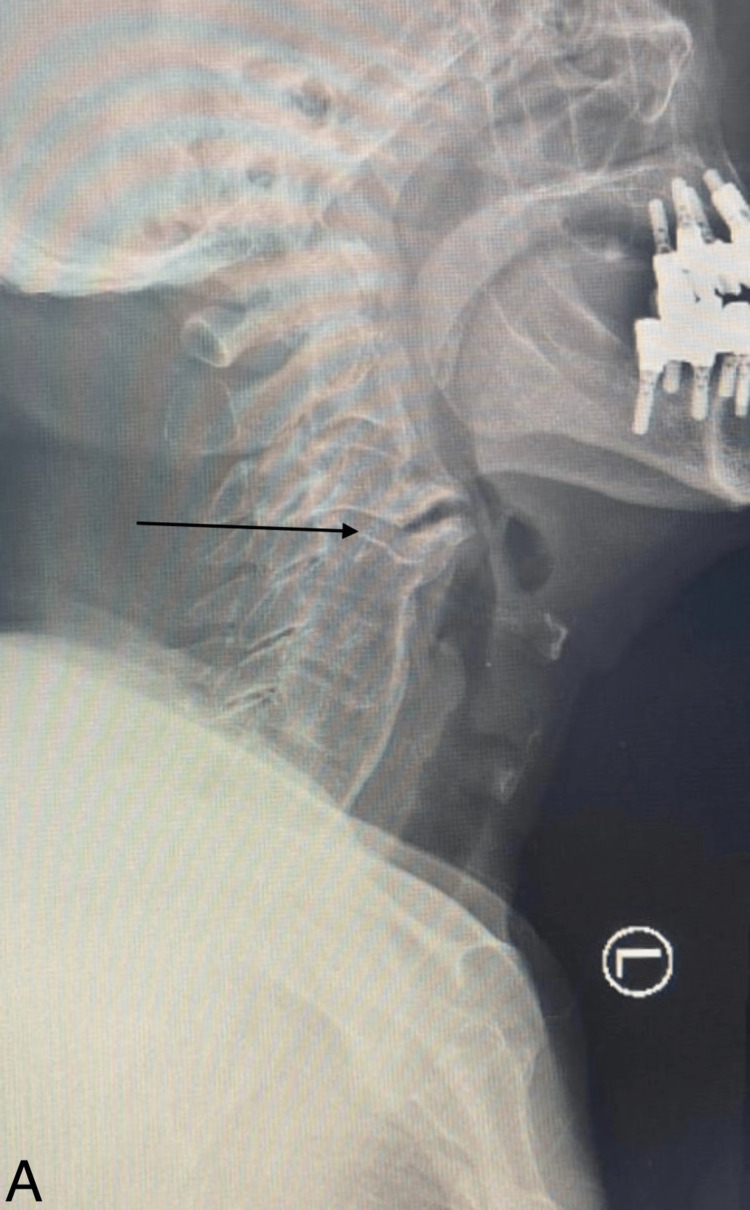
X-ray cervical spine; lateral view Showing anterior osteophytes at the C3-4 level
